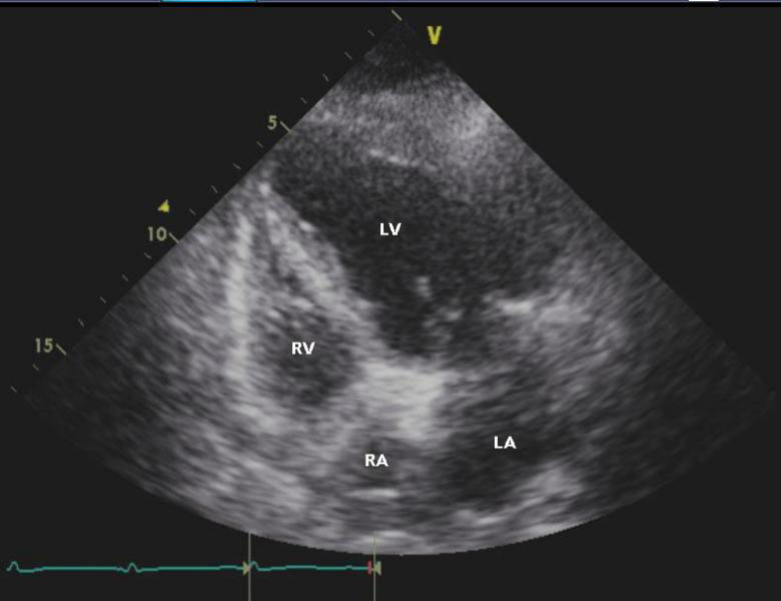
Echocardiogram in diastole (left ventricle systolic dysfunction).
